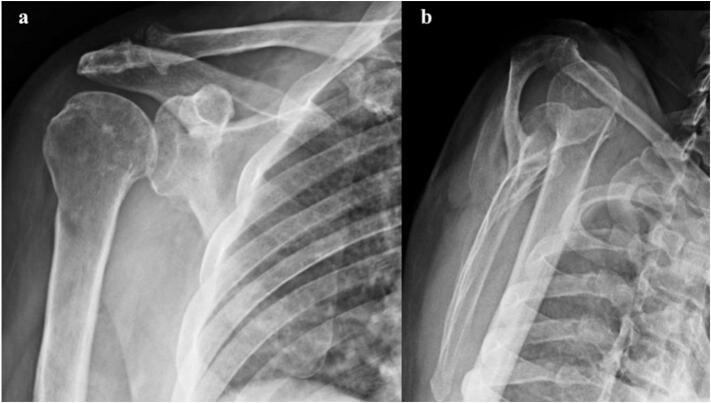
Radiographic study of the right shoulder. (a) AP view of the shoulder, no signs of significant arthritis can be observed; (b) Lateral view, superior and anterior subluxation of the shoulder is noticeable.
